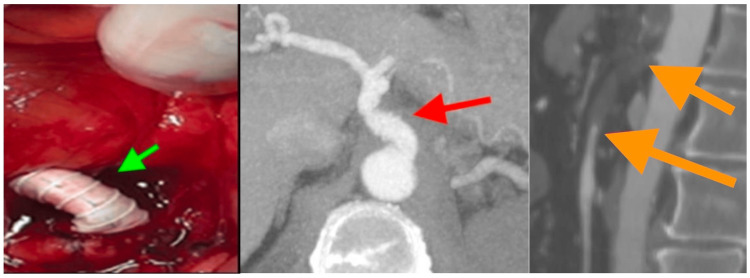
Intraoperative picture showing antegrade bypass from the aorta to the coeliac trunk (green arrow), and CTA of abdominal aorta one month postoperatively, showing patency of the aorta-coeliac bypass graft (red arrow), and recurrent thrombosis of the SMA (orange arrows).
